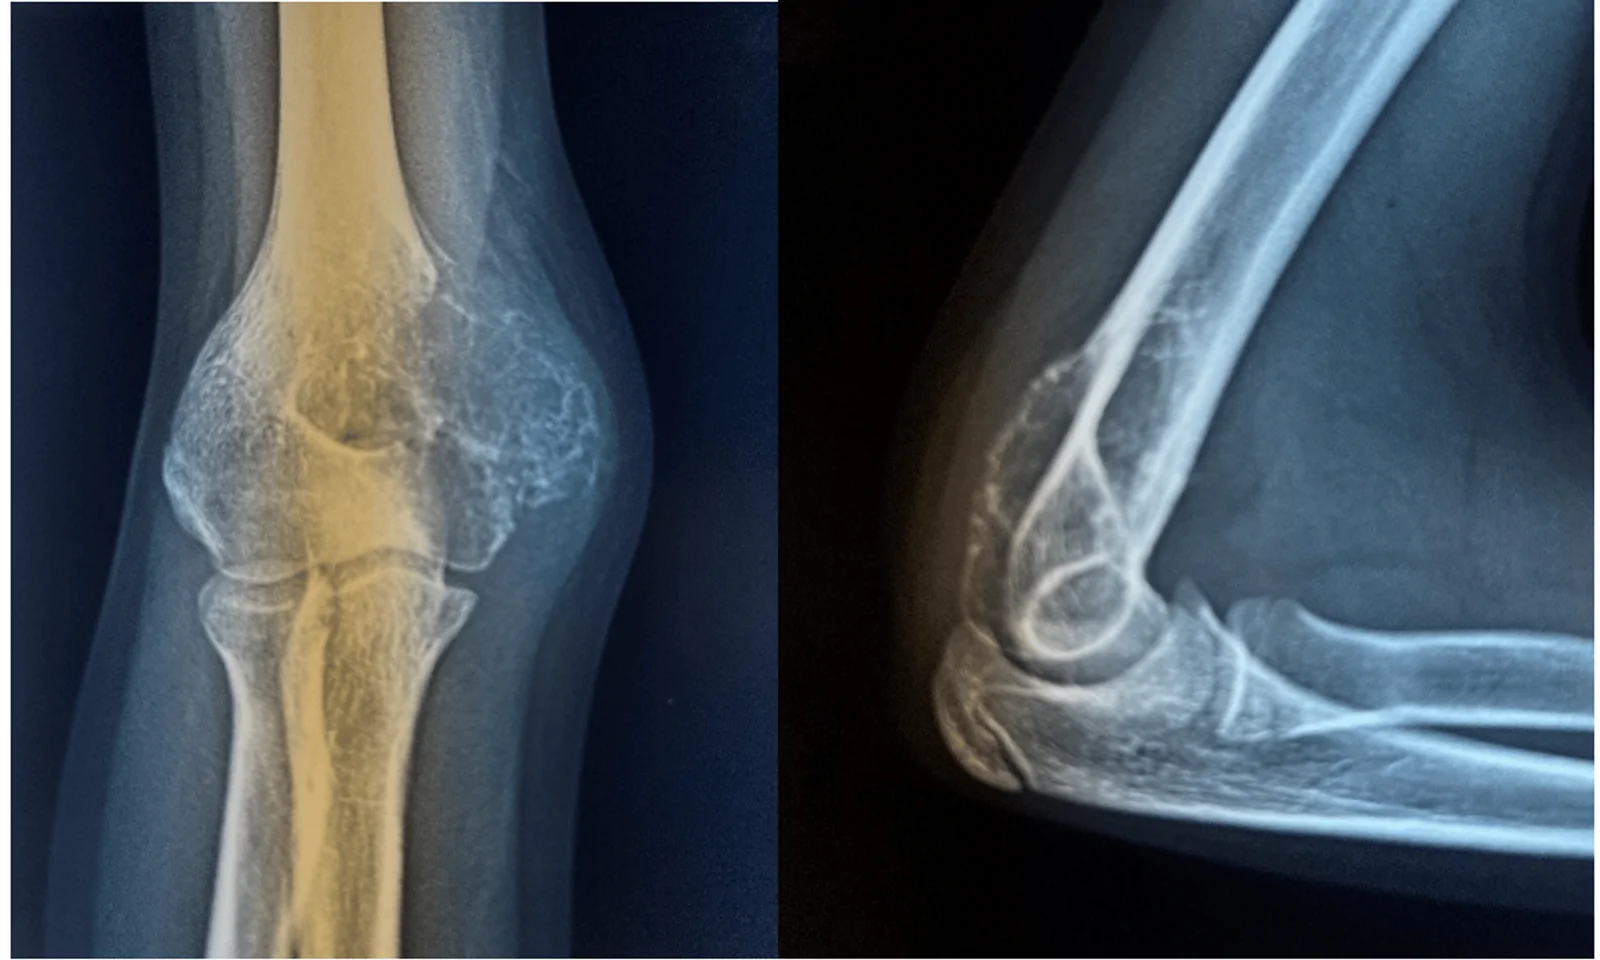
Anteroposterior and lateral plain radiographs of the right elbow demonstrating a large osteolytic lesion centered on the medial epicondyle of the distal humerus, with an associated periosteal reaction and cortical thinning.
